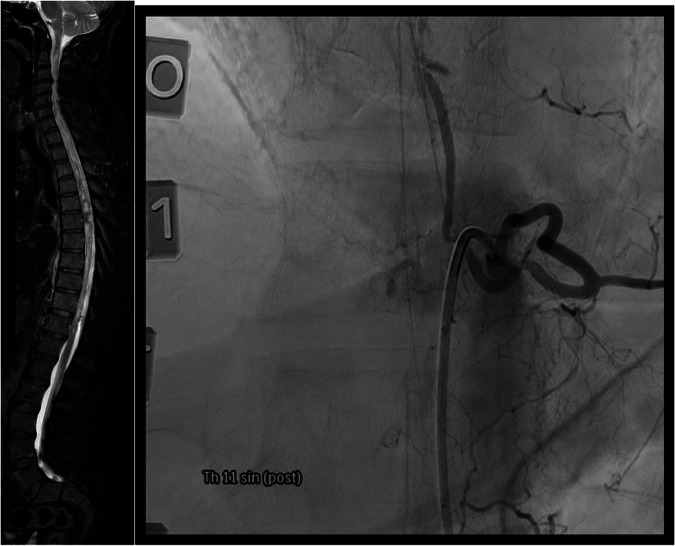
Similar to Fig. 1, Fig. 2 shows an MRI and angiography image of the same site, post-embolization. The MRI of the spine showing resolution of the lesions described previously and the angiography acquired subsequent to embolization, likewise, indicates clear resolution of the AVM.
